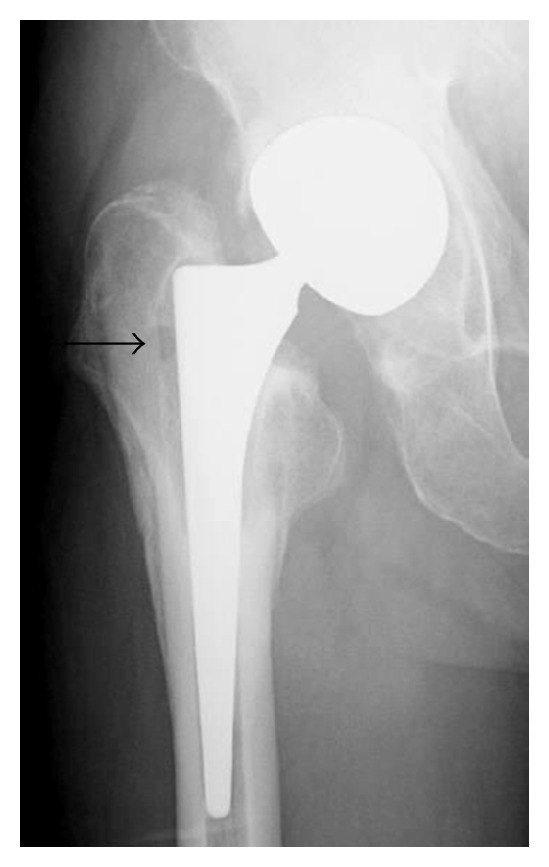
Radiograph showing a circular bone resorption (arrow).
